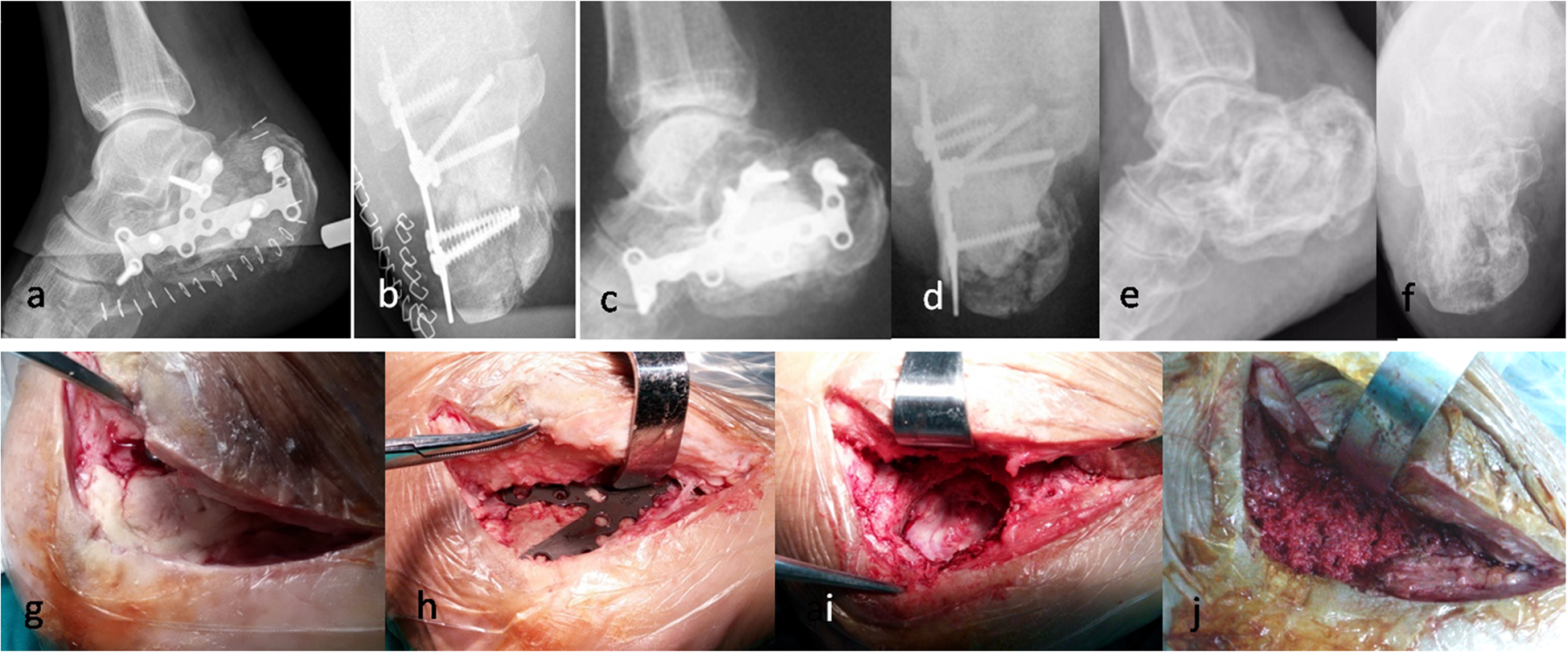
A 59-year-old male suffered calcaneus infection after fracture fixation, and was treated with irrigation, debridement, and retention of the implant combined with systemic and local antibiotic therapy. X-ray after fracture fixation showed the fracture was comminuted (a-b). 25 days after initial surgery, a thorough debridement was performed because of infection, two loose screws were removed, the plates were coated with antibiotic cement, and the bone defect was filled with antibiotic cement spacer (c-d). 8 months later, the bone cement(g) and the implants (h) were removed, and the cavity bone defect was filled with iliac bone grafts (i-j). At last follow- up, the bone was healed (e-f)
